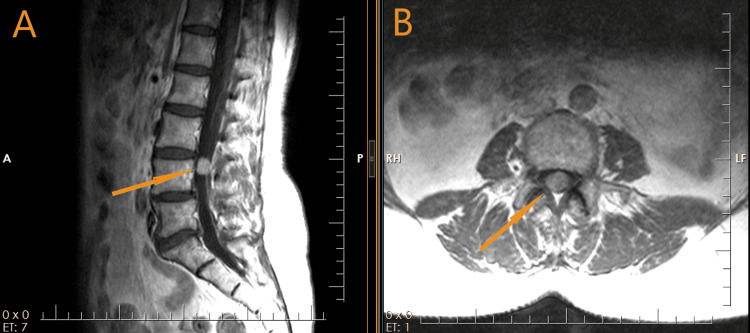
Sagittal (A) and axial (B) T1-weighted MRI with contrast enhancement showing an intradural homogeneous contrast-enhancing mass at the level of the L4 vertebrae (arrows). MRI: magnetic resonance imaging
